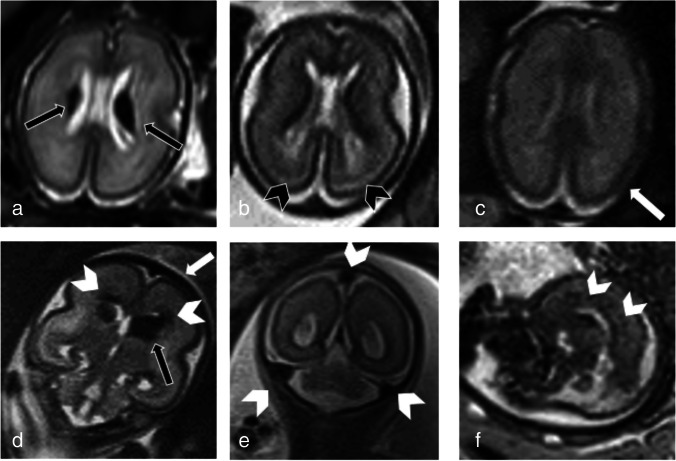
Twin Anemia-Polycythemia Sequence (TAPS) intracranial Magnetic Resonance (MRI) main findings in six different exemplificative cases: a) axial ss-FSE T2-weighted image from 24 week study showing bilateral intraventricular haemorrhage—IVH (black arrows), b) axial ss-FSE T2-weighted section from 22 week study showing bilateral para-trigonal blurred areas of higher T2-weighted signal (black arrow-heads), c) axial ss-FSE T2-weighted section from 25 week study showing cortical liquoral space obliteration due to brain swelling (arrow), d) coronal ss-FSE T2-weighted sequence from 22 week study showing germinal matrix hematoma (black arrow) with periventricular radial T2-weighted hypointensities (white arrow-head), brain swelling (white arrows), e–f) coronal and sagittal ss-FSE T2-weighted sections from 22 and 21 week study showing prominence of transverse and sagittal sinuses (arrow-heads in case in e), and deep medullary vein system (arrow-heads in case in f)
